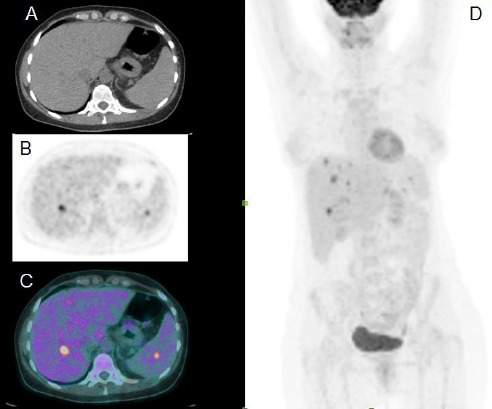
Axial CT (A), axial PET (B), and axial ^18^F-FDG PET/CT (C) images, showing increased FDG uptake in one hepatic (segment VI, SUV_max_=7.9) and one splenic (SUV_max_=5.2) lesion. Maximum intensity projection (MIP) image of the body (D) shows multiple areas of FDG uptake in the liver, with no other pathological uptakes
